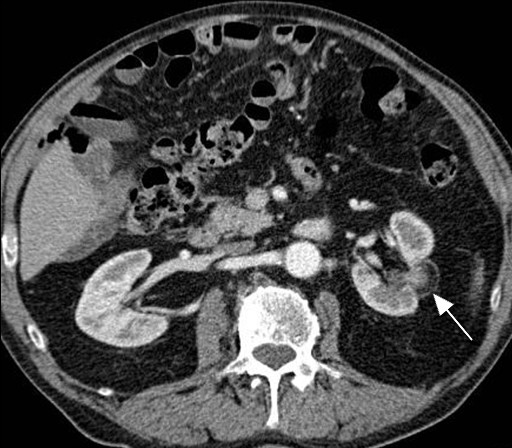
Axial section of contrast-enhanced CT of his left kidney after RFA shows the treated region (white arrow) with no evidence of residual or recurrent disease at two years after RFA.
